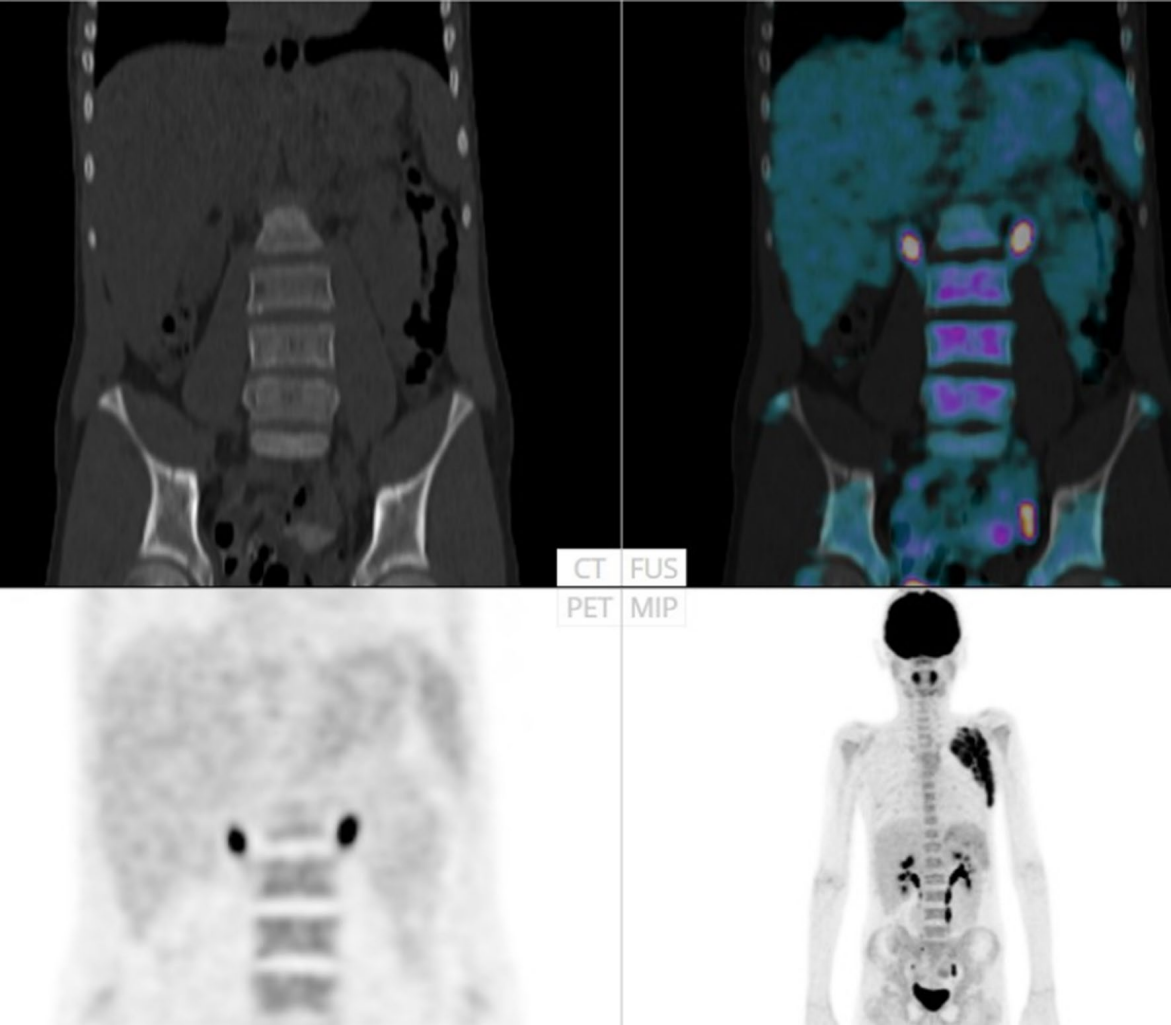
FDG-PET/CT: Example of a patient classified as having high diffuse bone marrow uptake (BMU) by the artificial intelligence-based method and by the manual method (SUV_index_ of 1,7 by both methods), while the majority (6/10) of the physicians classified this case as having low BMU.
